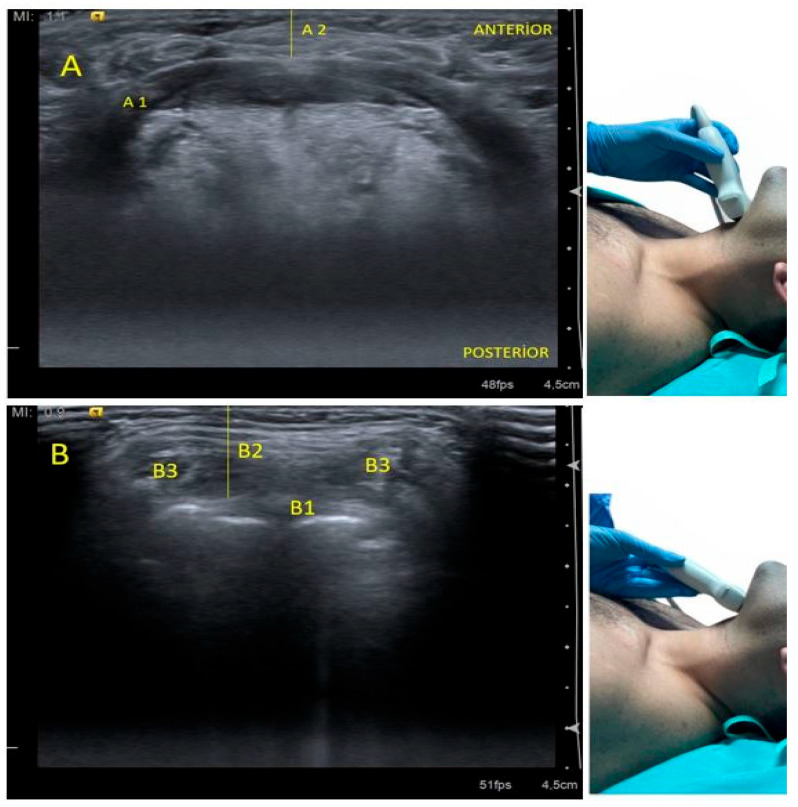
Representative ultrasonographic images demonstrating the measurement technique and anatomical landmarks used for focused upper airway ultrasonography. (A) Hyoid–skin distance measured at the anterior neck midline; A1, hyoid bone; A2, skin-to-hyoid distance. (B) Epiglottis–skin distance measured at the level of the thyrohyoid membrane; B1, epiglottis; B2, skin-to-epiglottis distance; B3, sternocleidomastoid muscle.
